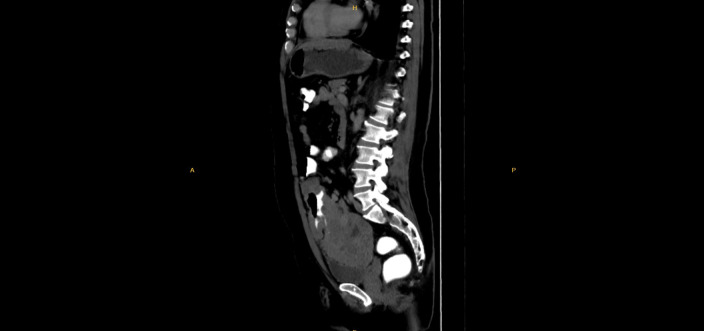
Contrast-enhanced CT showing sigmoid colon wall thickening.
